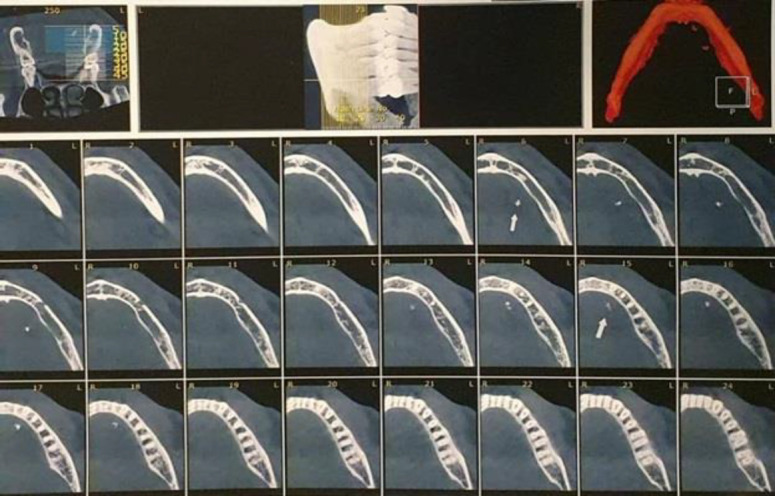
Computed tomography showing Wharton duct multiple stones
